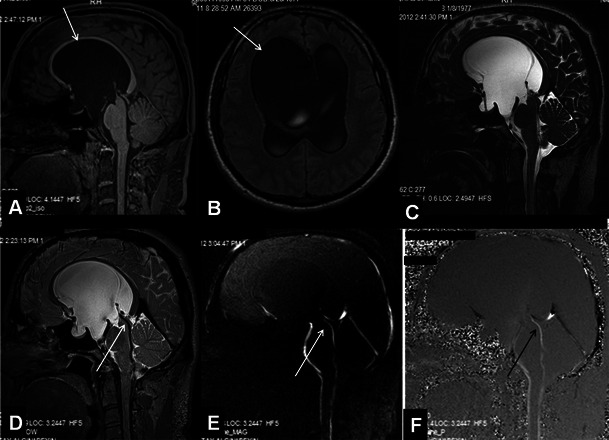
A 34-year-old male patient with intraventicular arachnoid cyst (AC) and hydrocephalus. Sagittal 3D-MPRAGE (A) and axial FLAIR (B) images show AC extending from the right lateral ventricle into the third ventricle (arrows). Sagittal heavily T2W 3D-SPACE image clearly shows the morphology of the AC and third ventricle (C). Sagittal 3D-SPACE with variant FA mode (D) and PC-MRI (E, F) images demonstrate the narrowed aqueduct and decreased aqueductal CSF flow (*arrows*)
